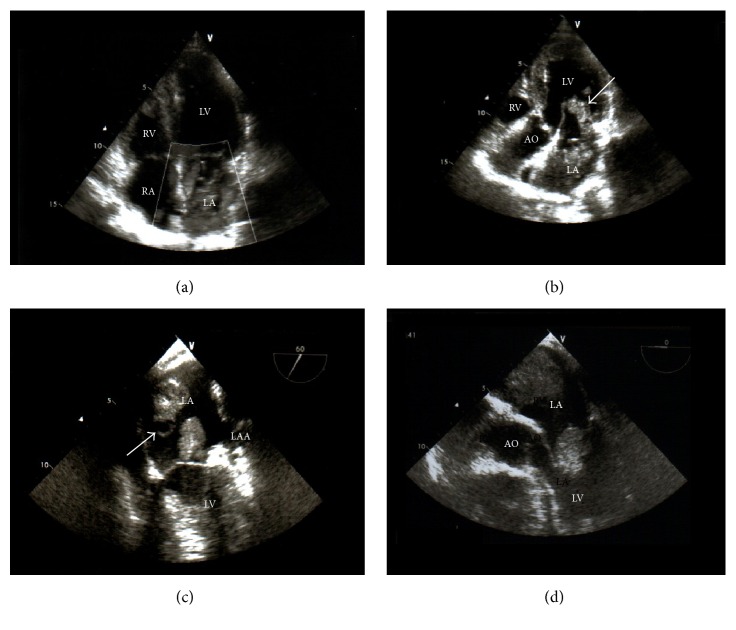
((a) and (b)) Transthoracic echocardiography showing massive left atrial invasion by a large multilobed mass, with protrusion through the mitral valve into the left ventricle during diastole (arrow). ((c) and (d)) Transesophageal echocardiography confirming left atrial invasion by a large mass entering the left ventricle in diastole and showing regions of cystic colliquation within the mass (arrow). AO, aorta; LA, left atrium; LAA, left atrial appendage; LV, left ventricle; RA, right atrium; RV, right ventricle.
